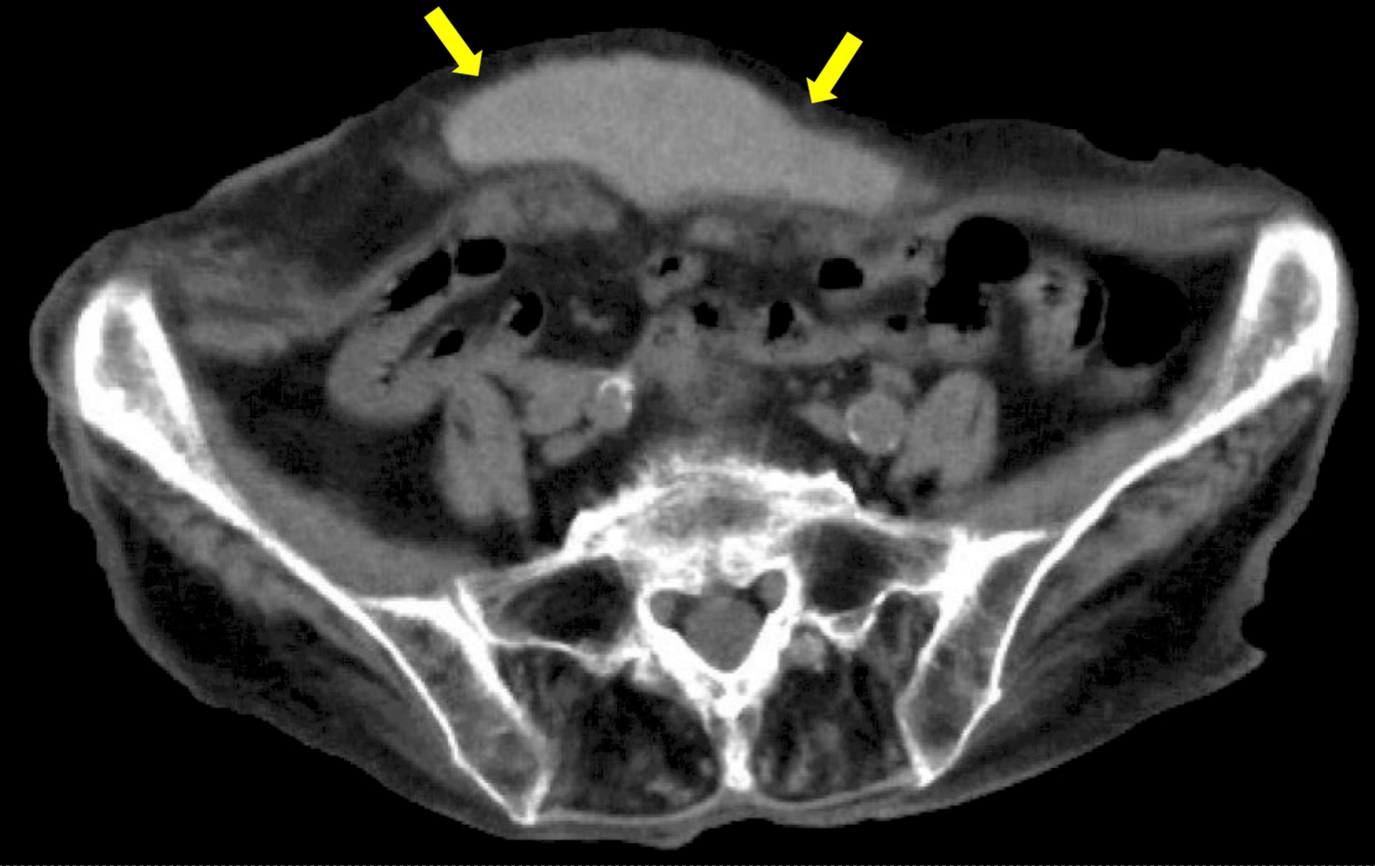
CT scan showing a rectus sheath hematoma on the fifth day.
